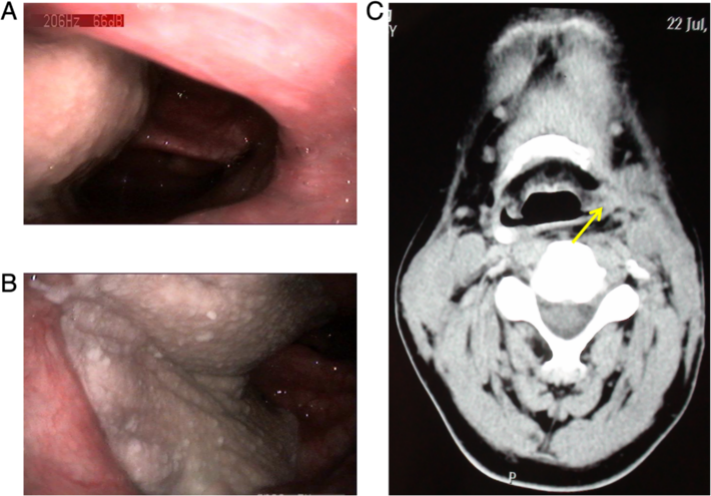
Electronic laryngoscopy and computed tomography scan reveal the free posterior tibial flap and hypopharynx in a patient one year after reconstruction. The yellow arrow indicates the posterior tibial flap. A and B: The posterior tibial flap and hypopharynx were revealed by electronic laryngoscopy through two different angles. C: The posterior tibial flap and hypopharynx were revealed by computed tomography.
